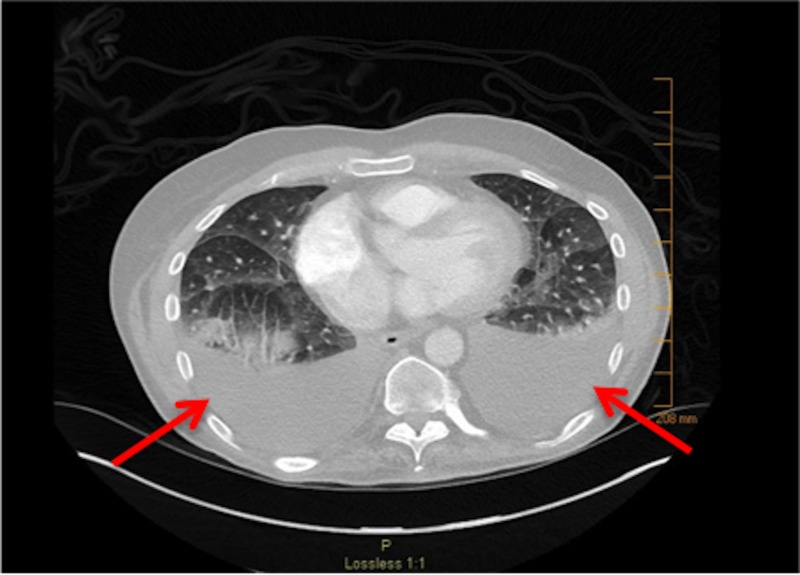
Bilateral pleural effusions CT scan showing bilateral pleural effusions (arrows). CT: computed tomography
